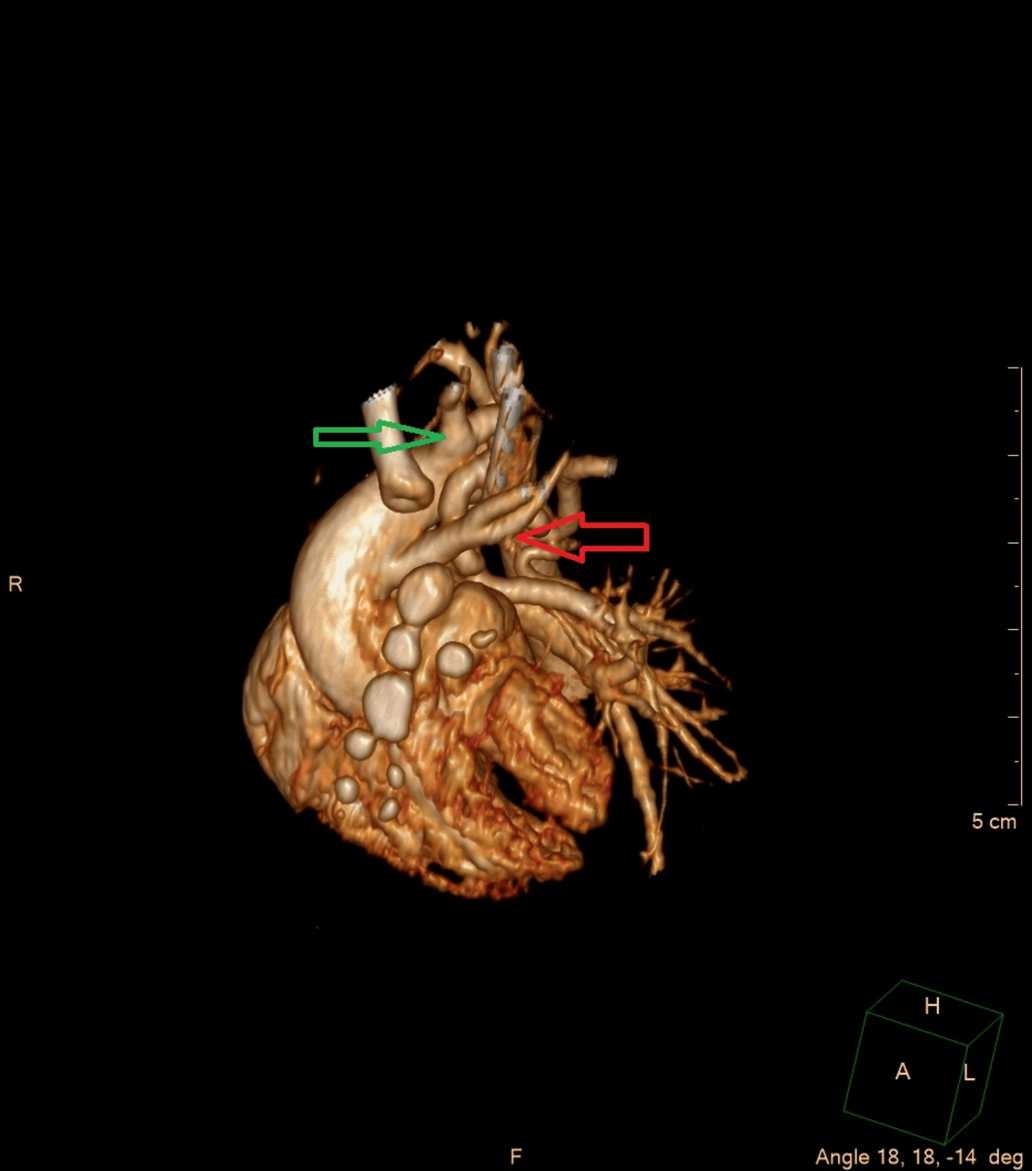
Image from the heart reconstruction created basing on the CT scans (volume-rendered images); front projection The red arrow points to the left common carotid artery, with an early division of the external and internal carotid arteries. The green arrow points to the right common carotid artery. Image credit: Created by the Pediatric Radiology Department team at the University Clinical Center of the Medical University of Warsaw.
